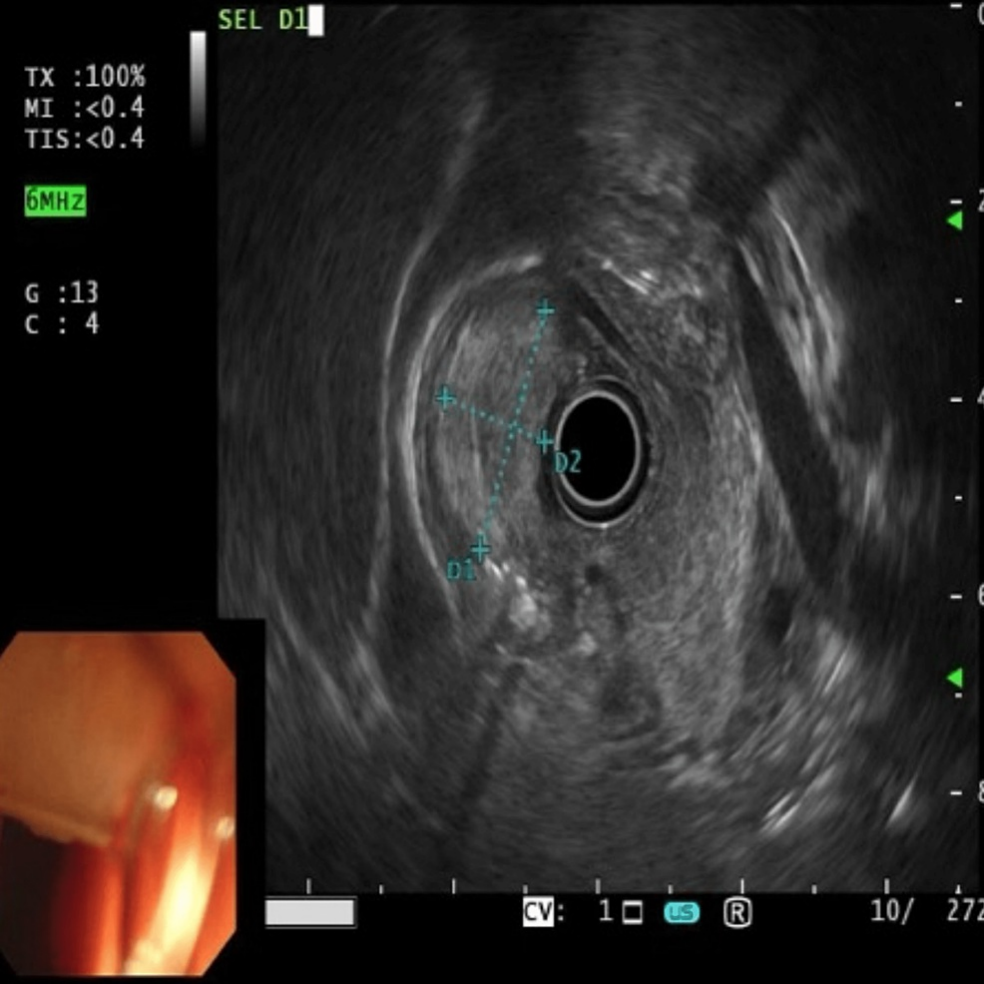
Endoscopic Ultrasound (EUS) showing a mass originated from 2nd mucosal layer
